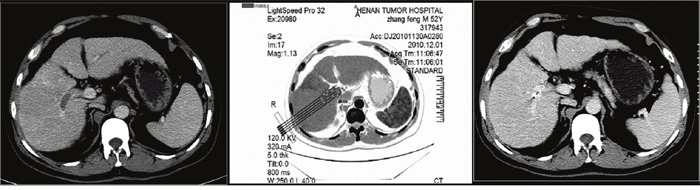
A 43-year-old man with hepatocellular carcinoma complicated with portal vein tumor thrombus in the right branch Enhanced computed tomography showed the portal vein tumor thrombus originated from the right branch of the portal vein. The image prior to iodine seed implantation showed the spatial distribution of seeds according to the treatment planning system. Computed tomography demonstrated that the portal vein tumor thrombus decreased in size and that the portal vein was recanalized two months after seed implantation.
